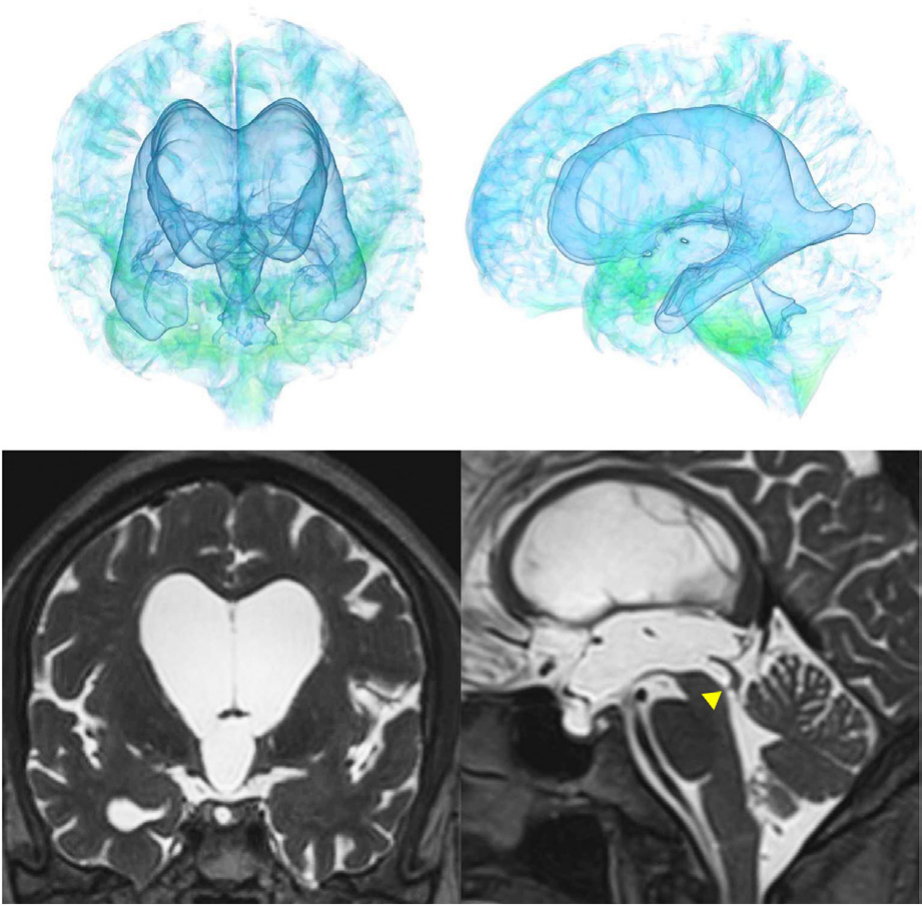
Case 2 diagnosed with adult-onset congenital NPH. A man aged 75 years old had huge lateral ventricles due to obstruction of the cerebral aqueduct by a membrane (yellow arrowhead) on sagittal thin-slice MRI.
